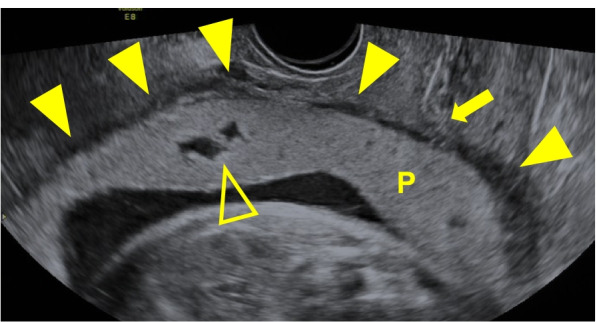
Transvaginal ultrasonography at 33 weeks’ gestation. A few small lacunae (hollow arrowhead) can be seen, but the retroplacental clear zone is preserved (solid arrowhead). The internal cervical os is indicated by an arrow. P: placenta
